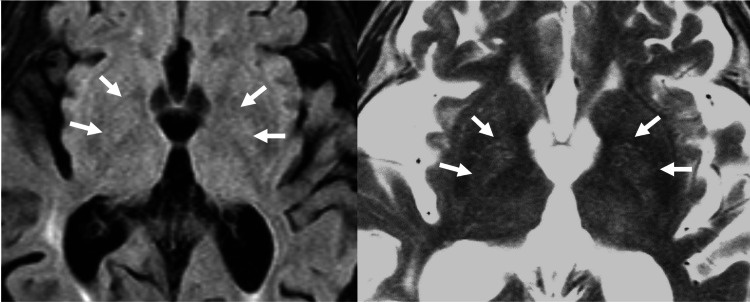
MR brain T1-weighted and T2-weighted MR brain imaging showed no acute infarct, hemorrhage, or intracranial mass effect. There was a bilateral signal abnormality (white arrows) in the globus pallidus. These imaging findings of the basal ganglia are bilateral and non-specific.
